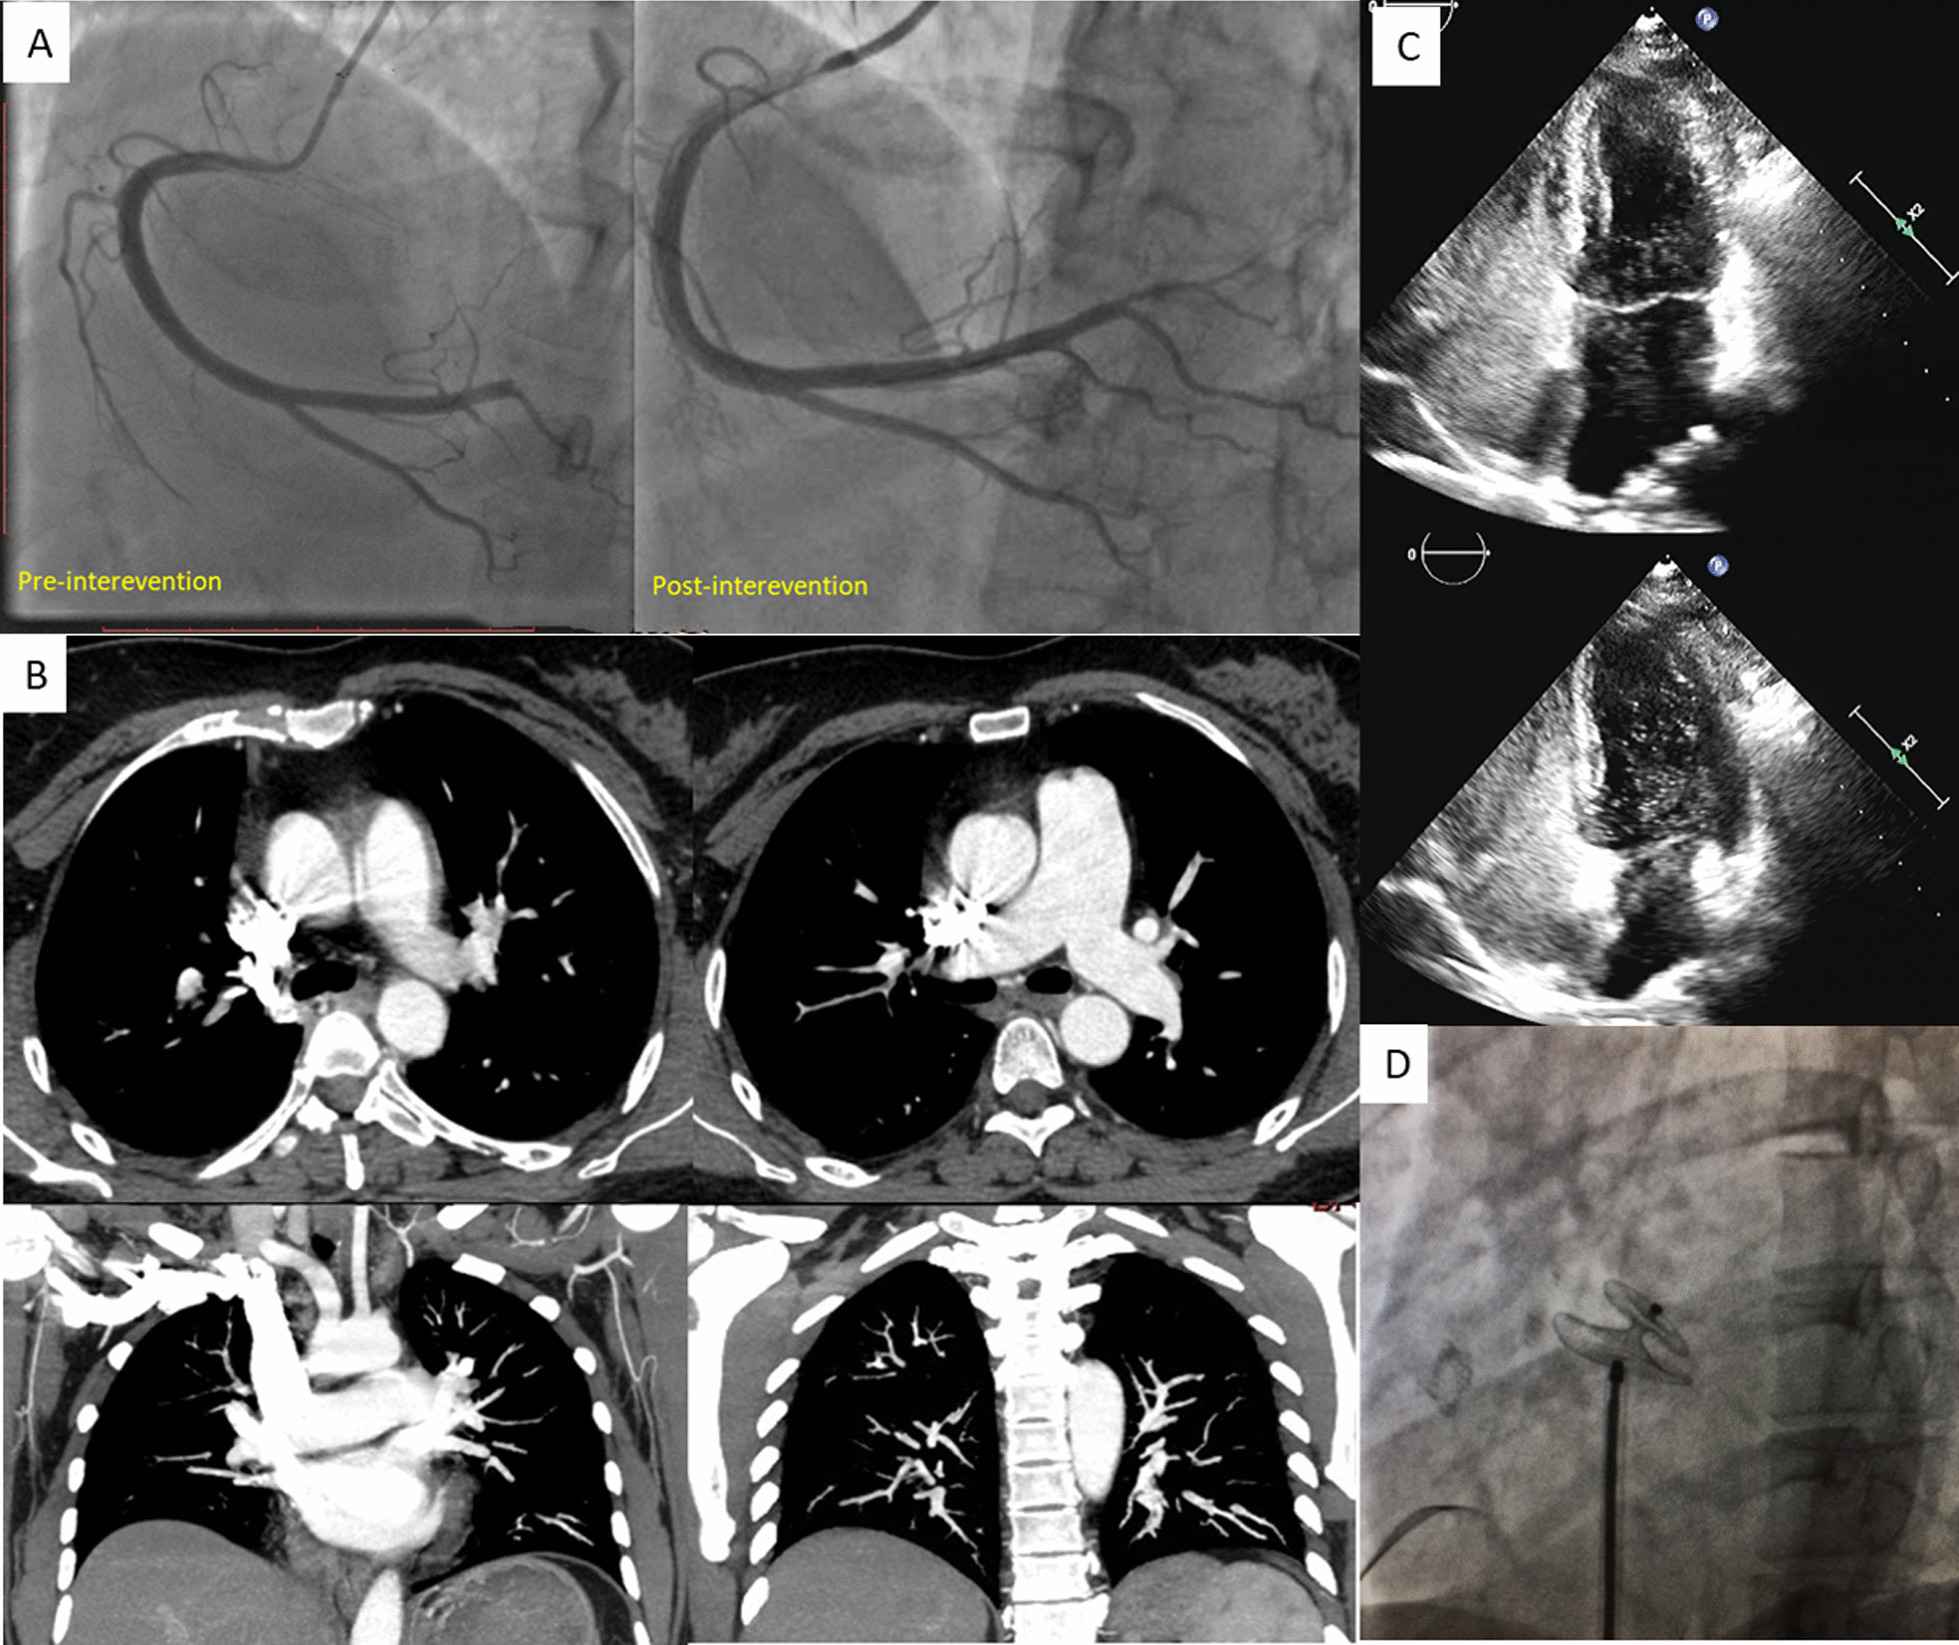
Procedure, post-procedure findings and management. A Post-intervention CAG showed no residual stenosis after embolus aspiration. B Pulmonary CTA at the one-month follow-up showed no residual emboli. C Bubble study showed > 10 bubbles crossed into LA in 1 cardiac cycle (upper: bubbles in LA and LV; lower: bubbles in LV). D PFO closure of this patient. CAG, cardioangiogram; CTA, CT angiography; PFO, patent foramen ovale
